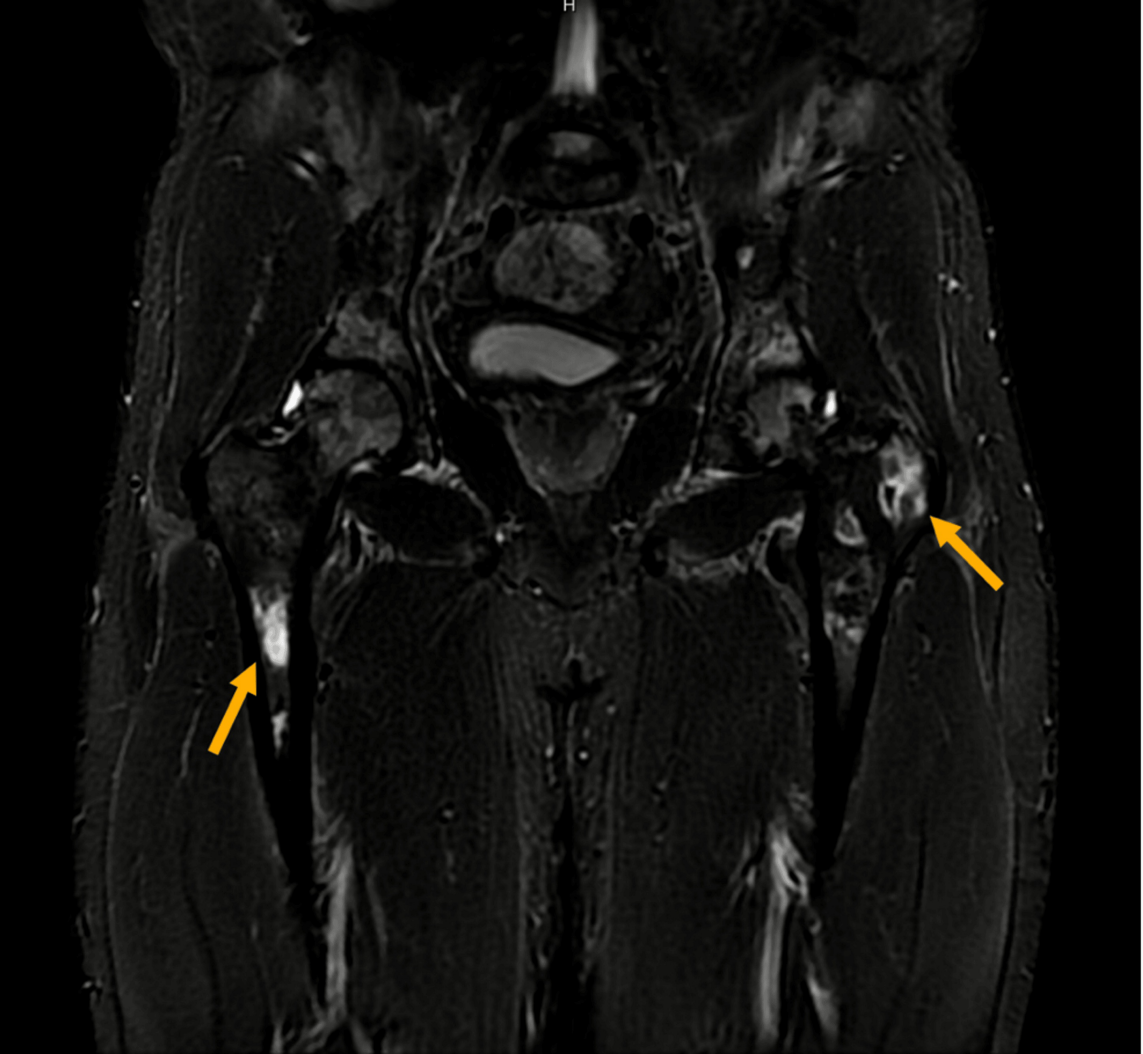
Coronal MRI demonstrating avascular necrosis of the femoral heads. Coronal short tau inversion recovery (STIR) MRI image shows bilateral femoral head hypointense areas (arrows), consistent with avascular necrosis.
